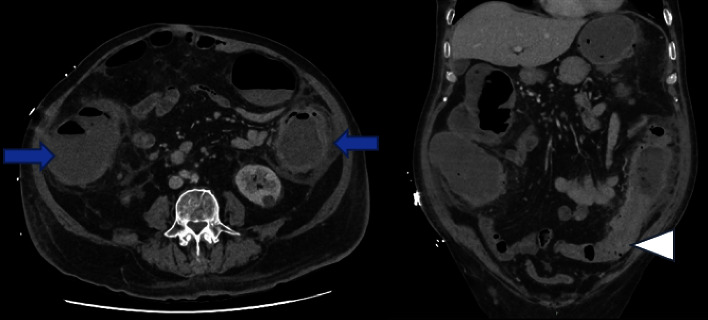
Abdominopelvic CT scans (axial and coronal views) demonstrating pancolitis (blue arrow) with a stricture in the mid-descending colon (white arrowhead) and dilated large bowel loops proximally.
